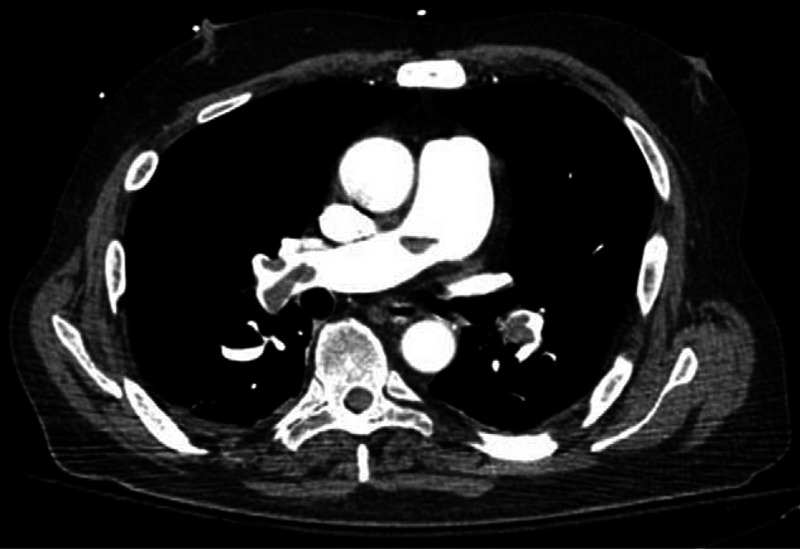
Computed tomography pulmonary angiogram shows multifocal filling defects in the pulmonary arterial system in keeping with acute bilateral pulmonary embolism.
